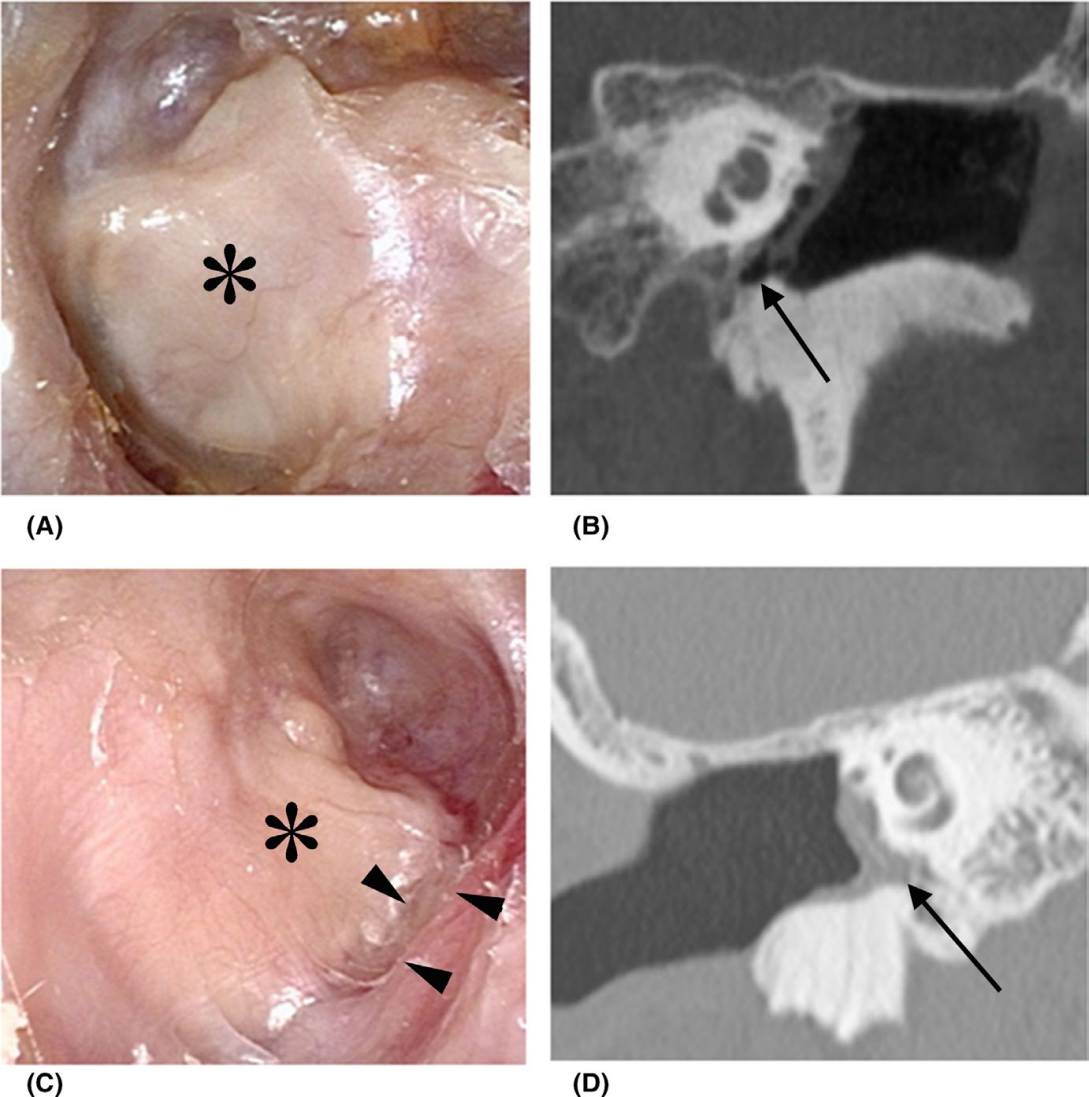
Postoperative findings in two representative eardrums at long‐term follow‐up. (A, B) Photographs of a left ear with an aerated mesotympanum (arrow) show a smooth reconstructed eardrum (asterisk) and tiny bumps between the eardrum and facial ridge at 9 years after surgery. (C, D) Computed tomography findings of a right ear without aeration (arrow) at 8.6 years after surgery show that the eardrum is slightly retracted (asterisk) and the sliced cartilage has become detached from the bony annulus (arrowhead)
